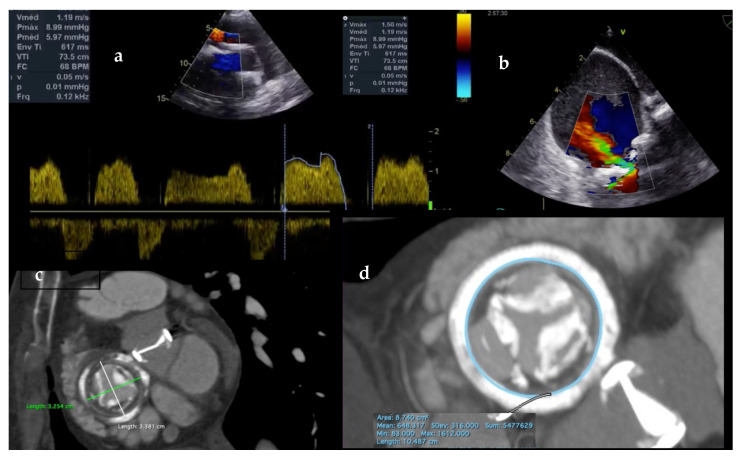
(a,b) Transesophageal echocardiography demonstrating RA–RV gradient and TV regurgitation. (c,d) Cardiac computed tomography showing bioprosthetic valve dimensions.
